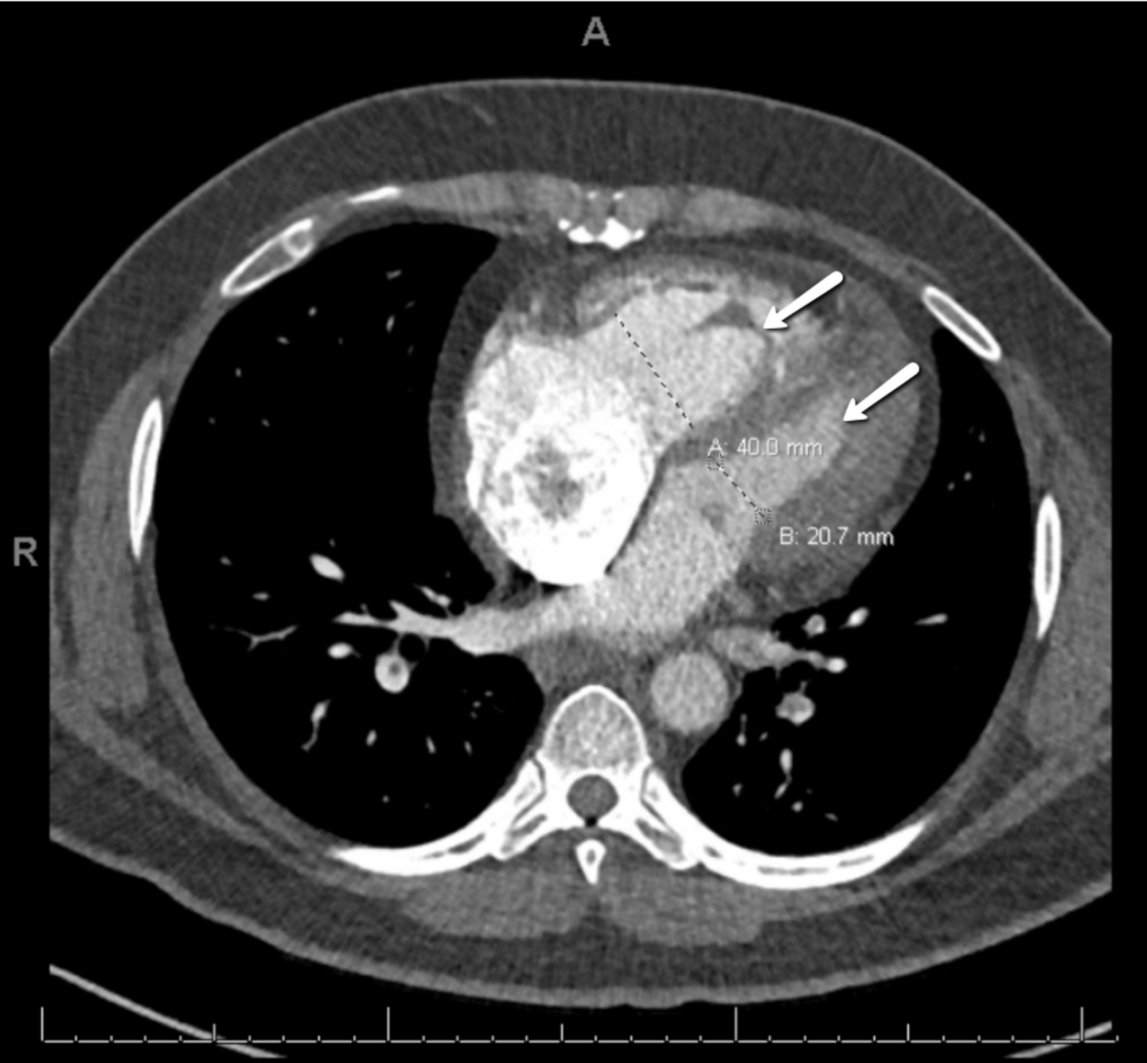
CT. Dilation of the right ventricle (40 mm) relative to the left ventricle (20.7 mm). The ratio of 2:1 suggests right heart strain.
